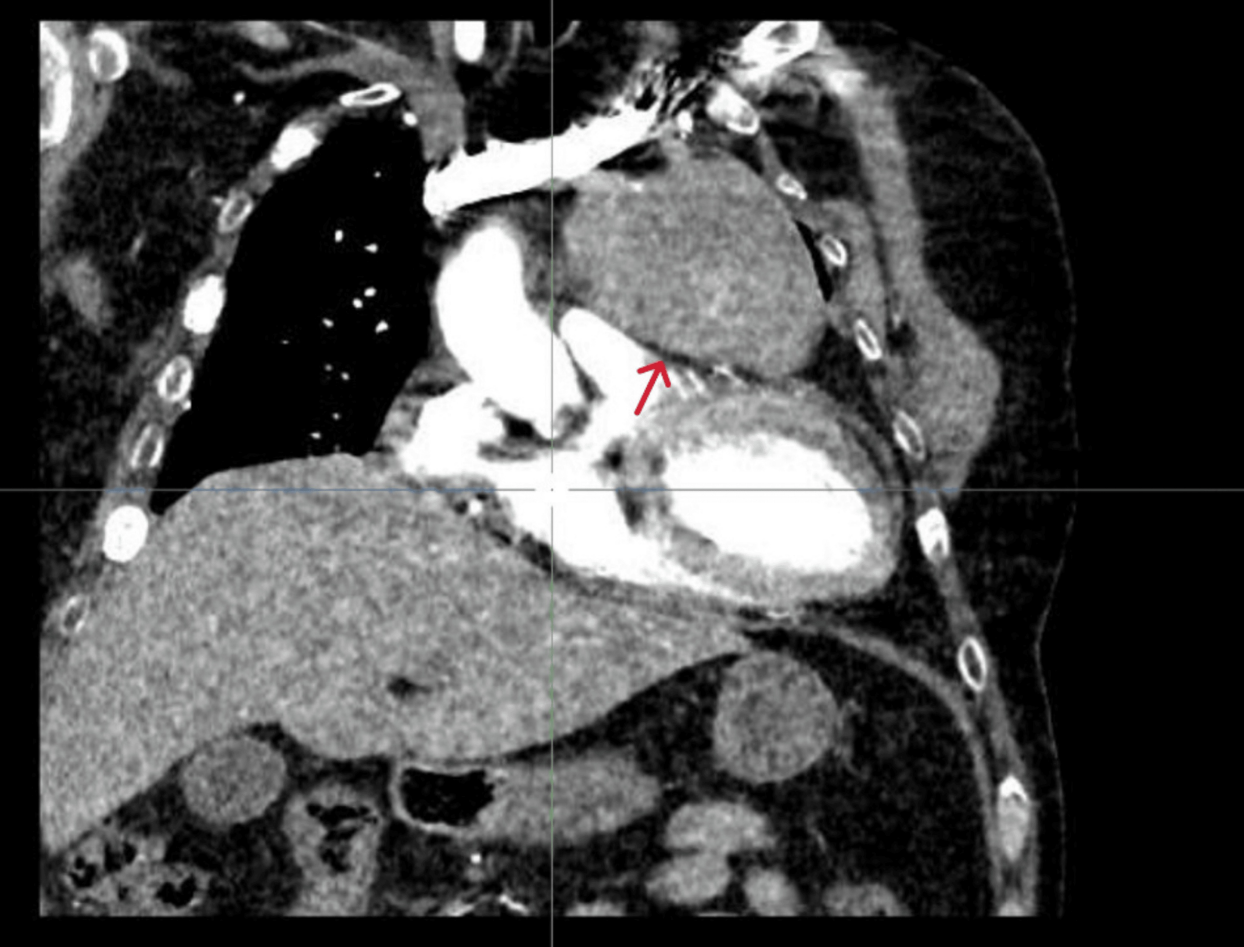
Coronal contrast-enhanced CT scan of the chest showing a large heterogeneous mediastinal mass extending towards the carina and involving adjacent mediastinal structures, including close contact with major vessels such as the ascending aorta and pulmonary artery. This suggests a locally advanced, unresectable tumor, likely of bronchopulmonary or lymphomatous origin (arrow)
